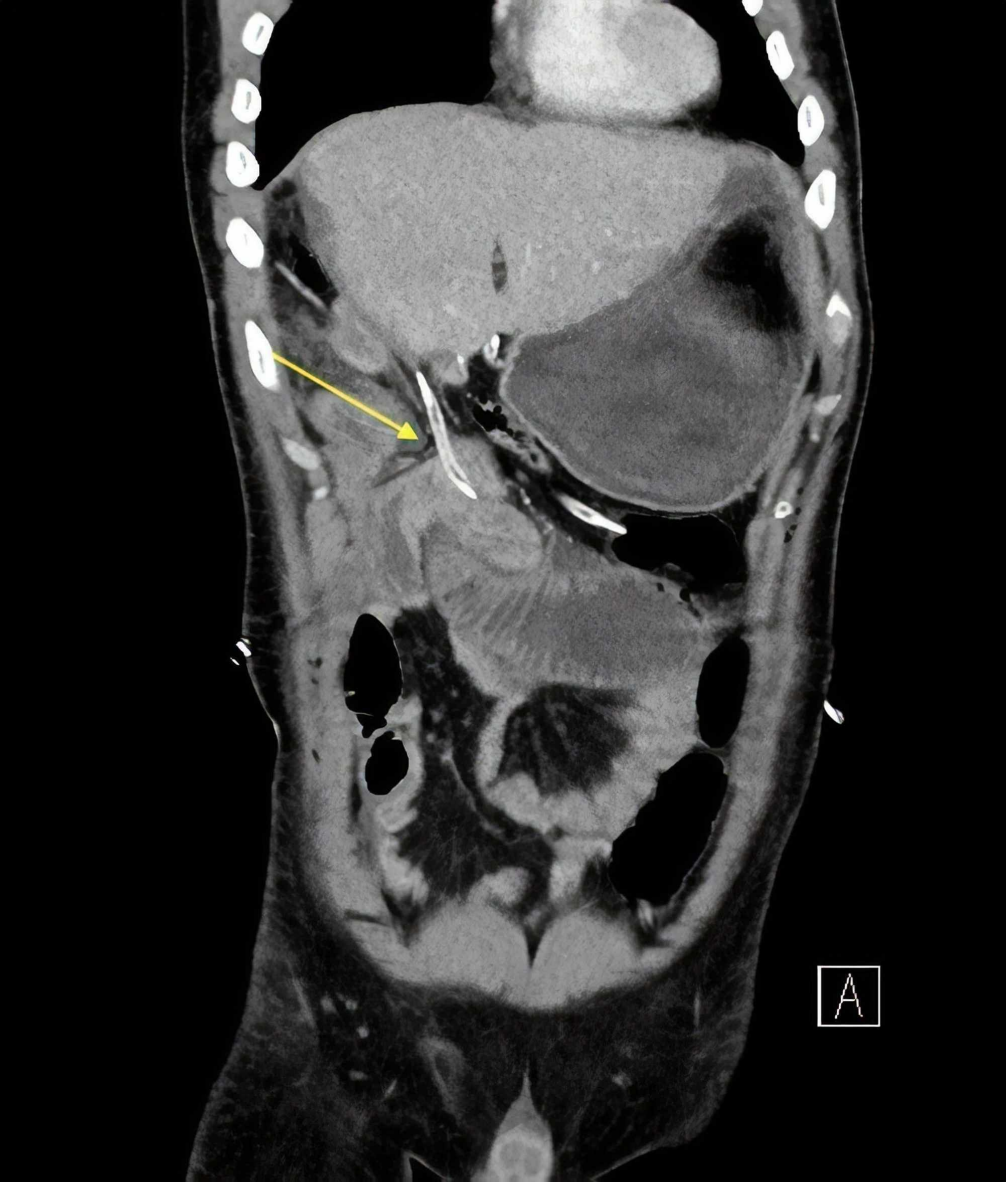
Coronal view of the CT scan showing the trajectory of the culprit drain. The stomach is distended as a result of the obstruction.
